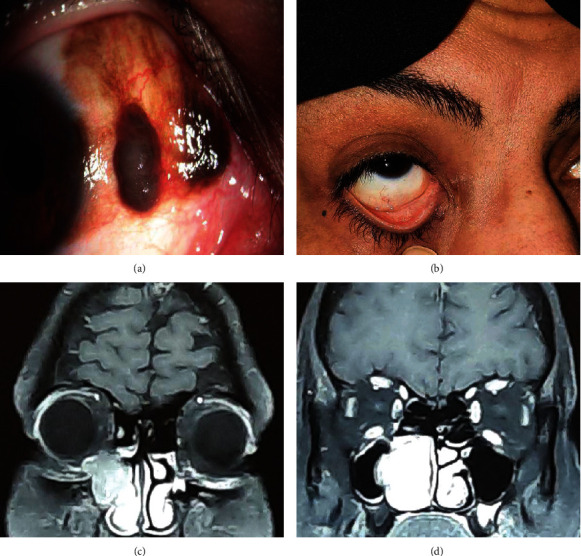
(a) Photoslit of the patient shows two separated lobulated nodular melanotic masses. (b) There is no clinical ocular surface, punctal, medial canthal, and caruncular involvement at the time of intranasal mass diagnosis. (c, d) Orbital and paranasal sinus MRI, coronal section, demonstrating intranasal moderately enhancing mass involving the right-sided nasal cavity and inferomedial part of the right orbit.
